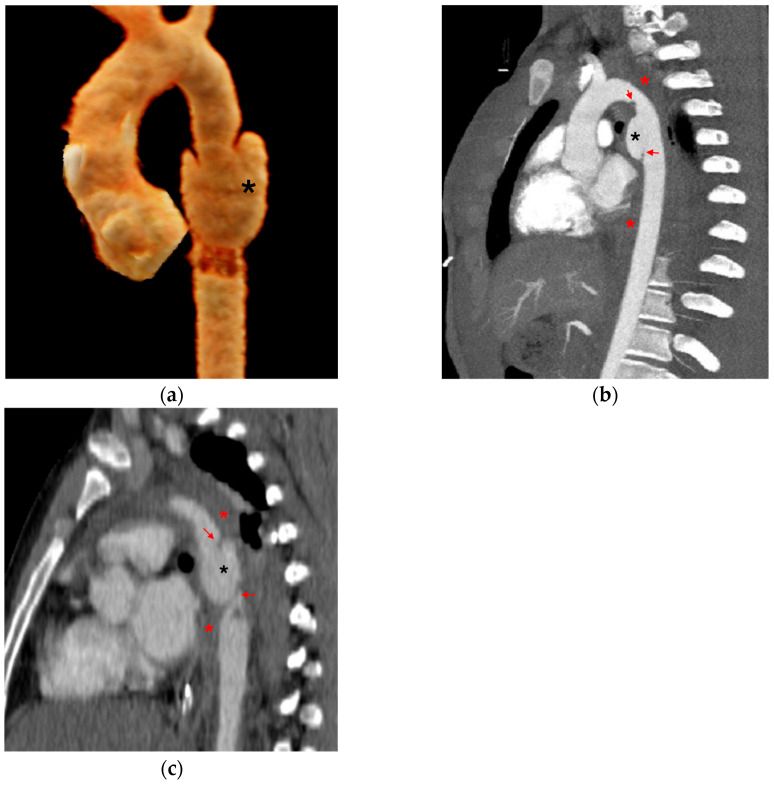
Pseudoaneurysm. (a) 3D volume-rendered and (b,c) sagittal CTA images show focal dilatation (black asterisks) and intimal disruption (red arrows) along with a periaortic hematoma (red asterisks).
